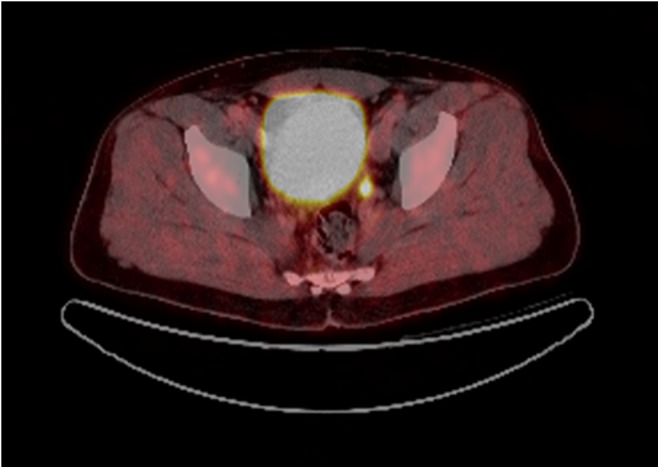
Transversal plane (18)F-DOPA-PET/CT of norepinephrine-producing tumor recurrence localized in the left seminal vesicle.
